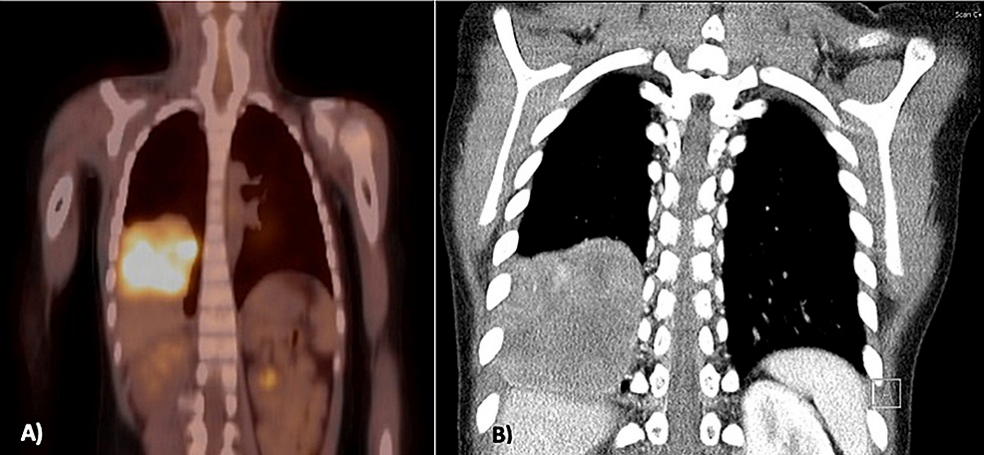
Imaging at the first relapse A) PET scan, B) CT scan
